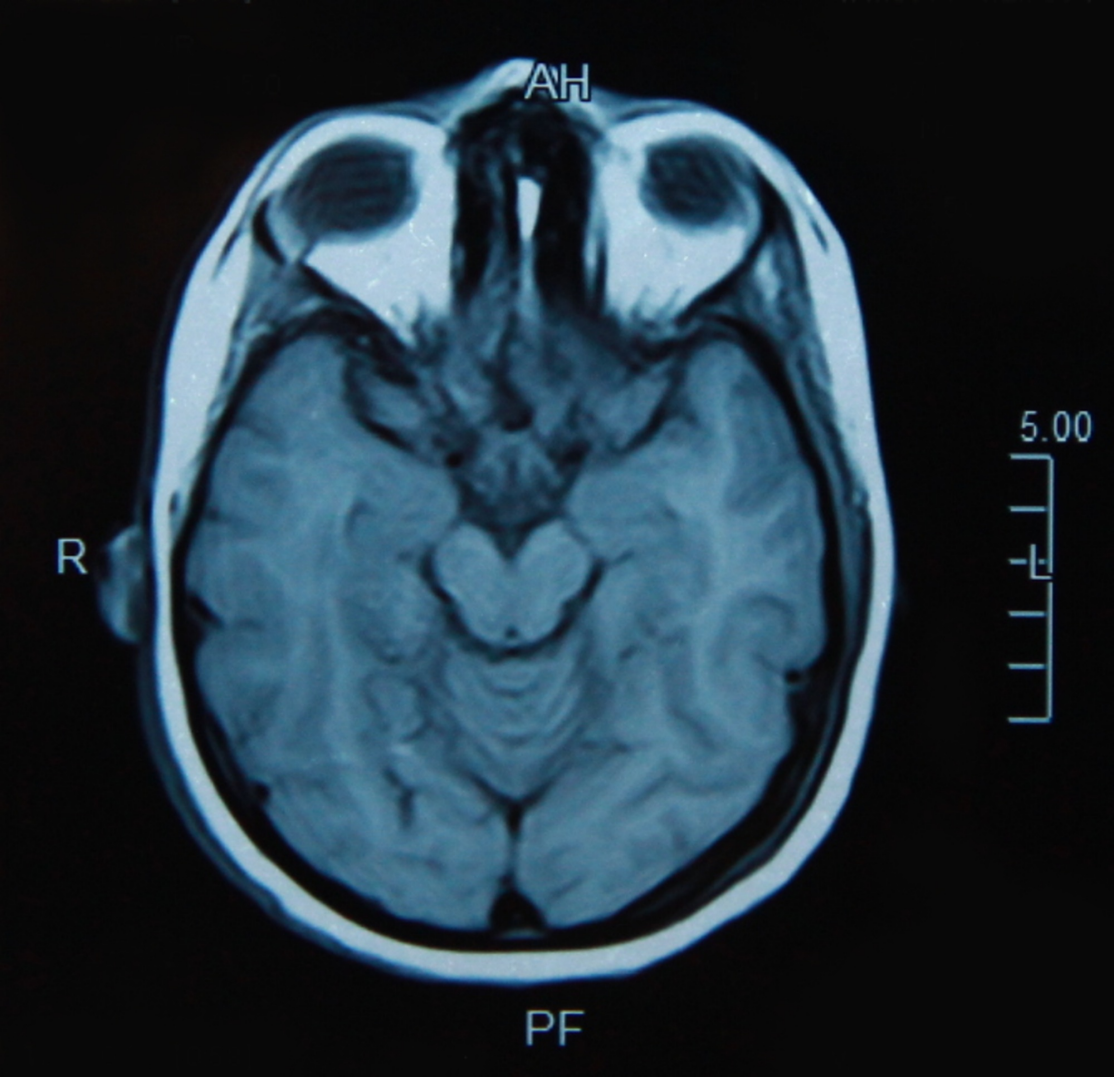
Magnetic resonance imaging (MRI) of the brain, T1-weighted image, axial view showing normal brain parenchyma without any radiological changes
